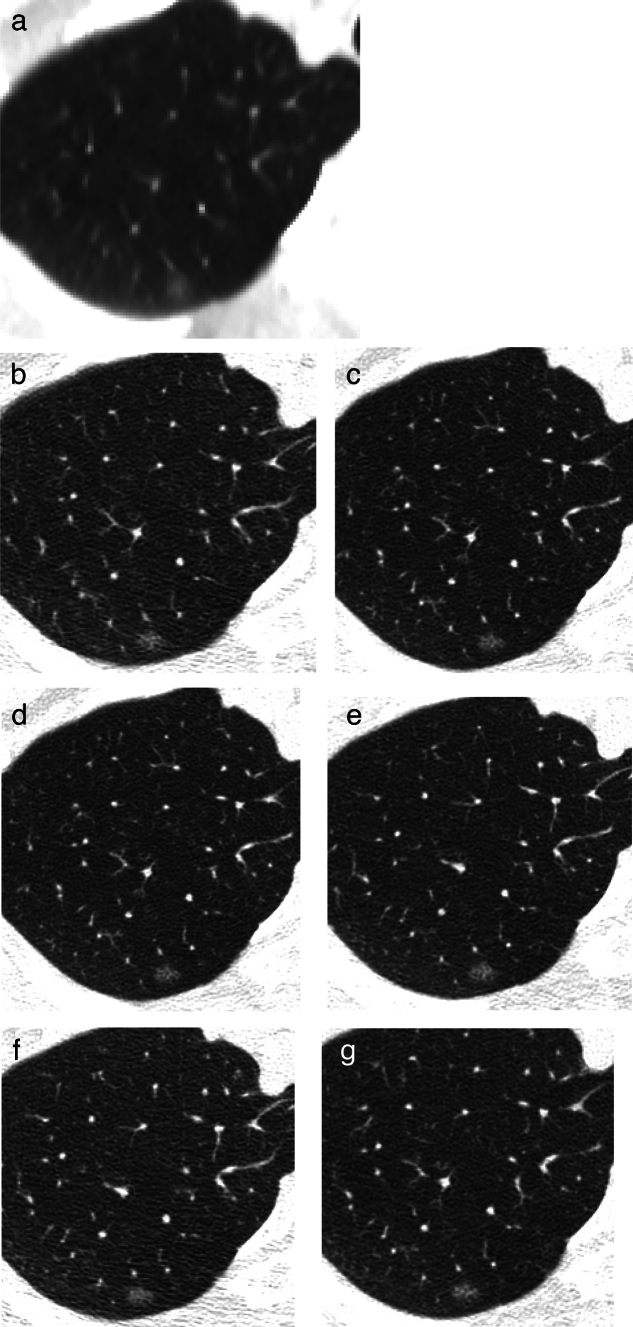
Ground-glass nodule with no temporal change. a Low-dose CT screening image (a) Shooting conditions: CTDIvol = 0.6 mGy. Slice thickness: 2.5 mm; sequential approximation reconstruction (model-based iterative reconstruction: MBIR) (WW WL) = (1,200–, 600). The low-dose CT screening image (a) reveals a faint nodule measuring 6.3 × 5.8 mm in the right upper lobe. b–g Thin-section CT (axial images) 2 weeks after screening (b), 3 months later (c), 12 months later (d), 24 months later (e), 48 months later (f), 60 months later (g); slice thickness: 0.625 mm (WW WL) = (1,200–, 600). After the detection of an average diameter of ≥ 6 mm in the low-dose CT screening, a thin-section CT was performed 2 weeks later. The CT revealed a ground-glass nodule with total dimensions of 6.2 mm. Subsequent thin-section CT scans conducted at 3, 12, 24, 48, and 60 months showed no changes in the appearance of the nodule
